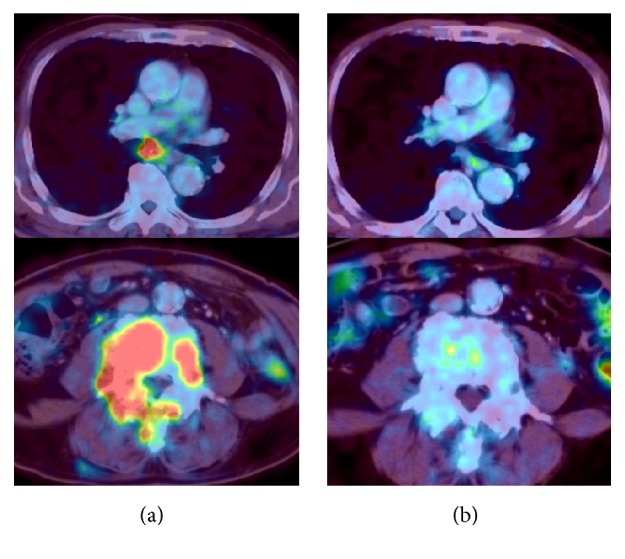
PET-CT showing (a) lymph node metastasis near the bifurcation of trachea and lumbar vertebral metastasis before sorafenib rechallenge therapy and (b) that the metastatic lesion vanished 5 months after the rechallenge therapy.
